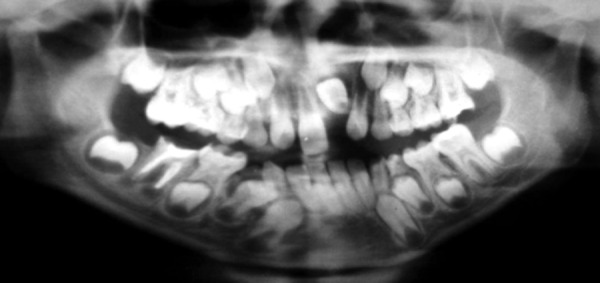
Preoperative OPG radiograph
